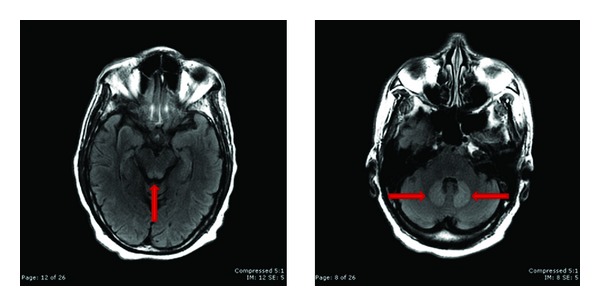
FLAIR sequences at the level of the cerebellum and the brainstem demonstrate hyperintense T2 signal in the bilateral dentate nuclei and inferior colliculi. No diffusion signal abnormality was identified (not shown).
